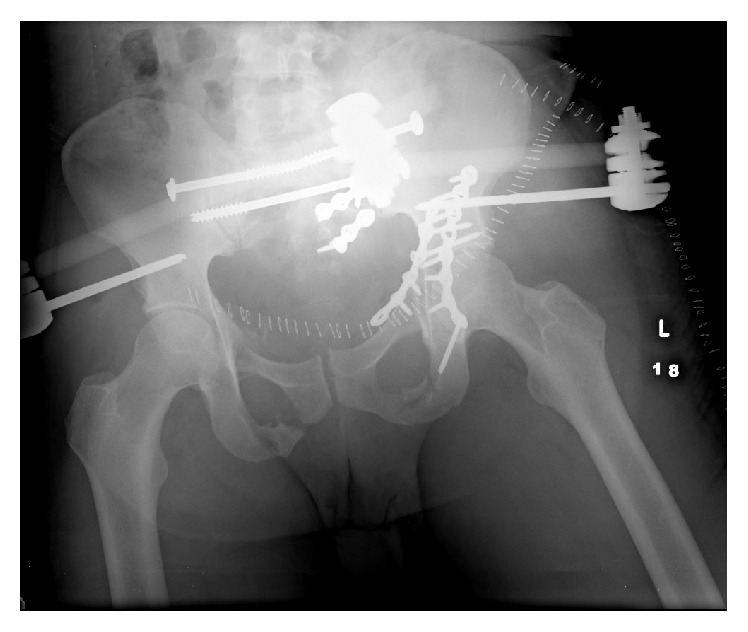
AP pelvis radiograph status after stabilization of the pelvis.
